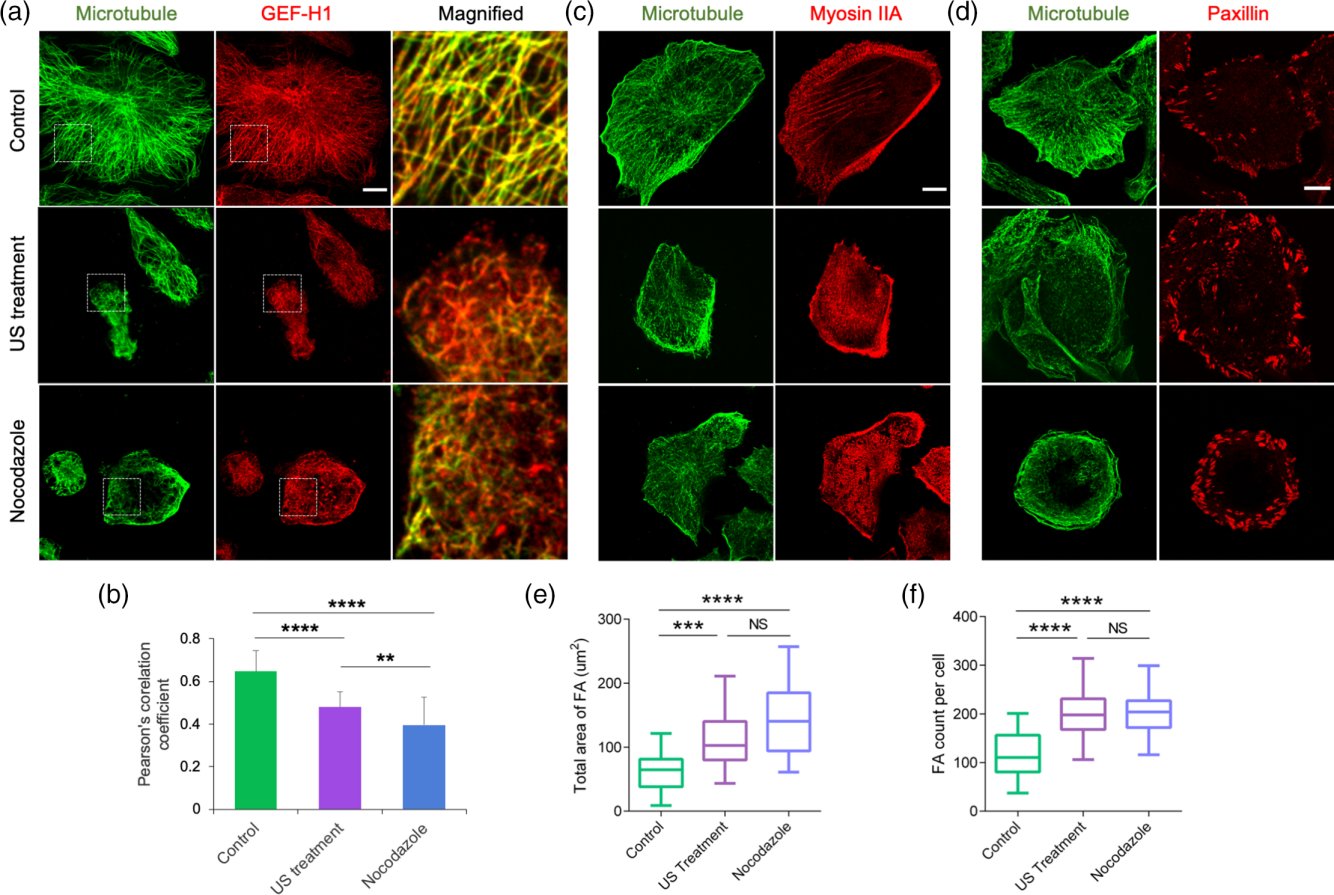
Ultrasound promotes GEF‐H1 release, myosin‐IIA assembly, and adhesion size. (a) Immunofluorescent images showing spatial distribution of microtubules and GEF‐H1 in the control, ultrasound‐ and nocodazole‐treated MDA‐MB‐231 tumor cells. (b) Pearson's correlation coefficient displaying the extent of microtubule and GEF‐H1 colocalization under different experimental condition, *n* = 25 cells from two independent experiments. (c) Representative images showing spatial distribution of microtubules and myosin IIA in the control, ultrasound‐ and nocodazole‐treated cells. (d) Representative images displaying microtubules and paxillin in the control, ultrasound‐ and nocodazole‐treated cells. (e,f) Box‐and‐whisker plot indicating total area of adhesions per cell and number of adhesions per cell respectively in the control, ultrasound‐ and nocodazole‐treated cells, *n* = 25 cells. Whiskers extend from the minimum to maximum values, the box extends from the 25th to the 75th percentile and the line within the box represents the median. *p* < 0.01, p* < 0.001, *p* < 0.0001. In all image panels, scale bar: 10 μm
